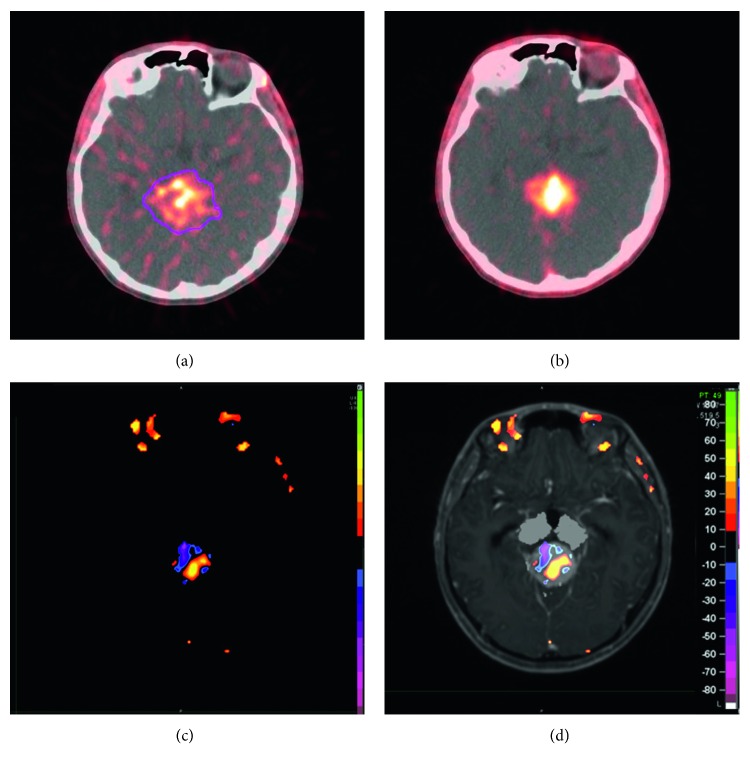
Pre-CK PET/CT image (a), post-CK PET/CT image (b), subtraction (c) of PET image, and fused PET/MRI images (d) of a male patient, 21 years old, diagnosed with germ cell tumor. Color bar shown in (d) corresponds to radioactivity changing ratio. The change in tracer uptake from pre-CK (a) to post-CK (b) is visualized by the subtraction of PET images (c) and PET/MRI images (d).
